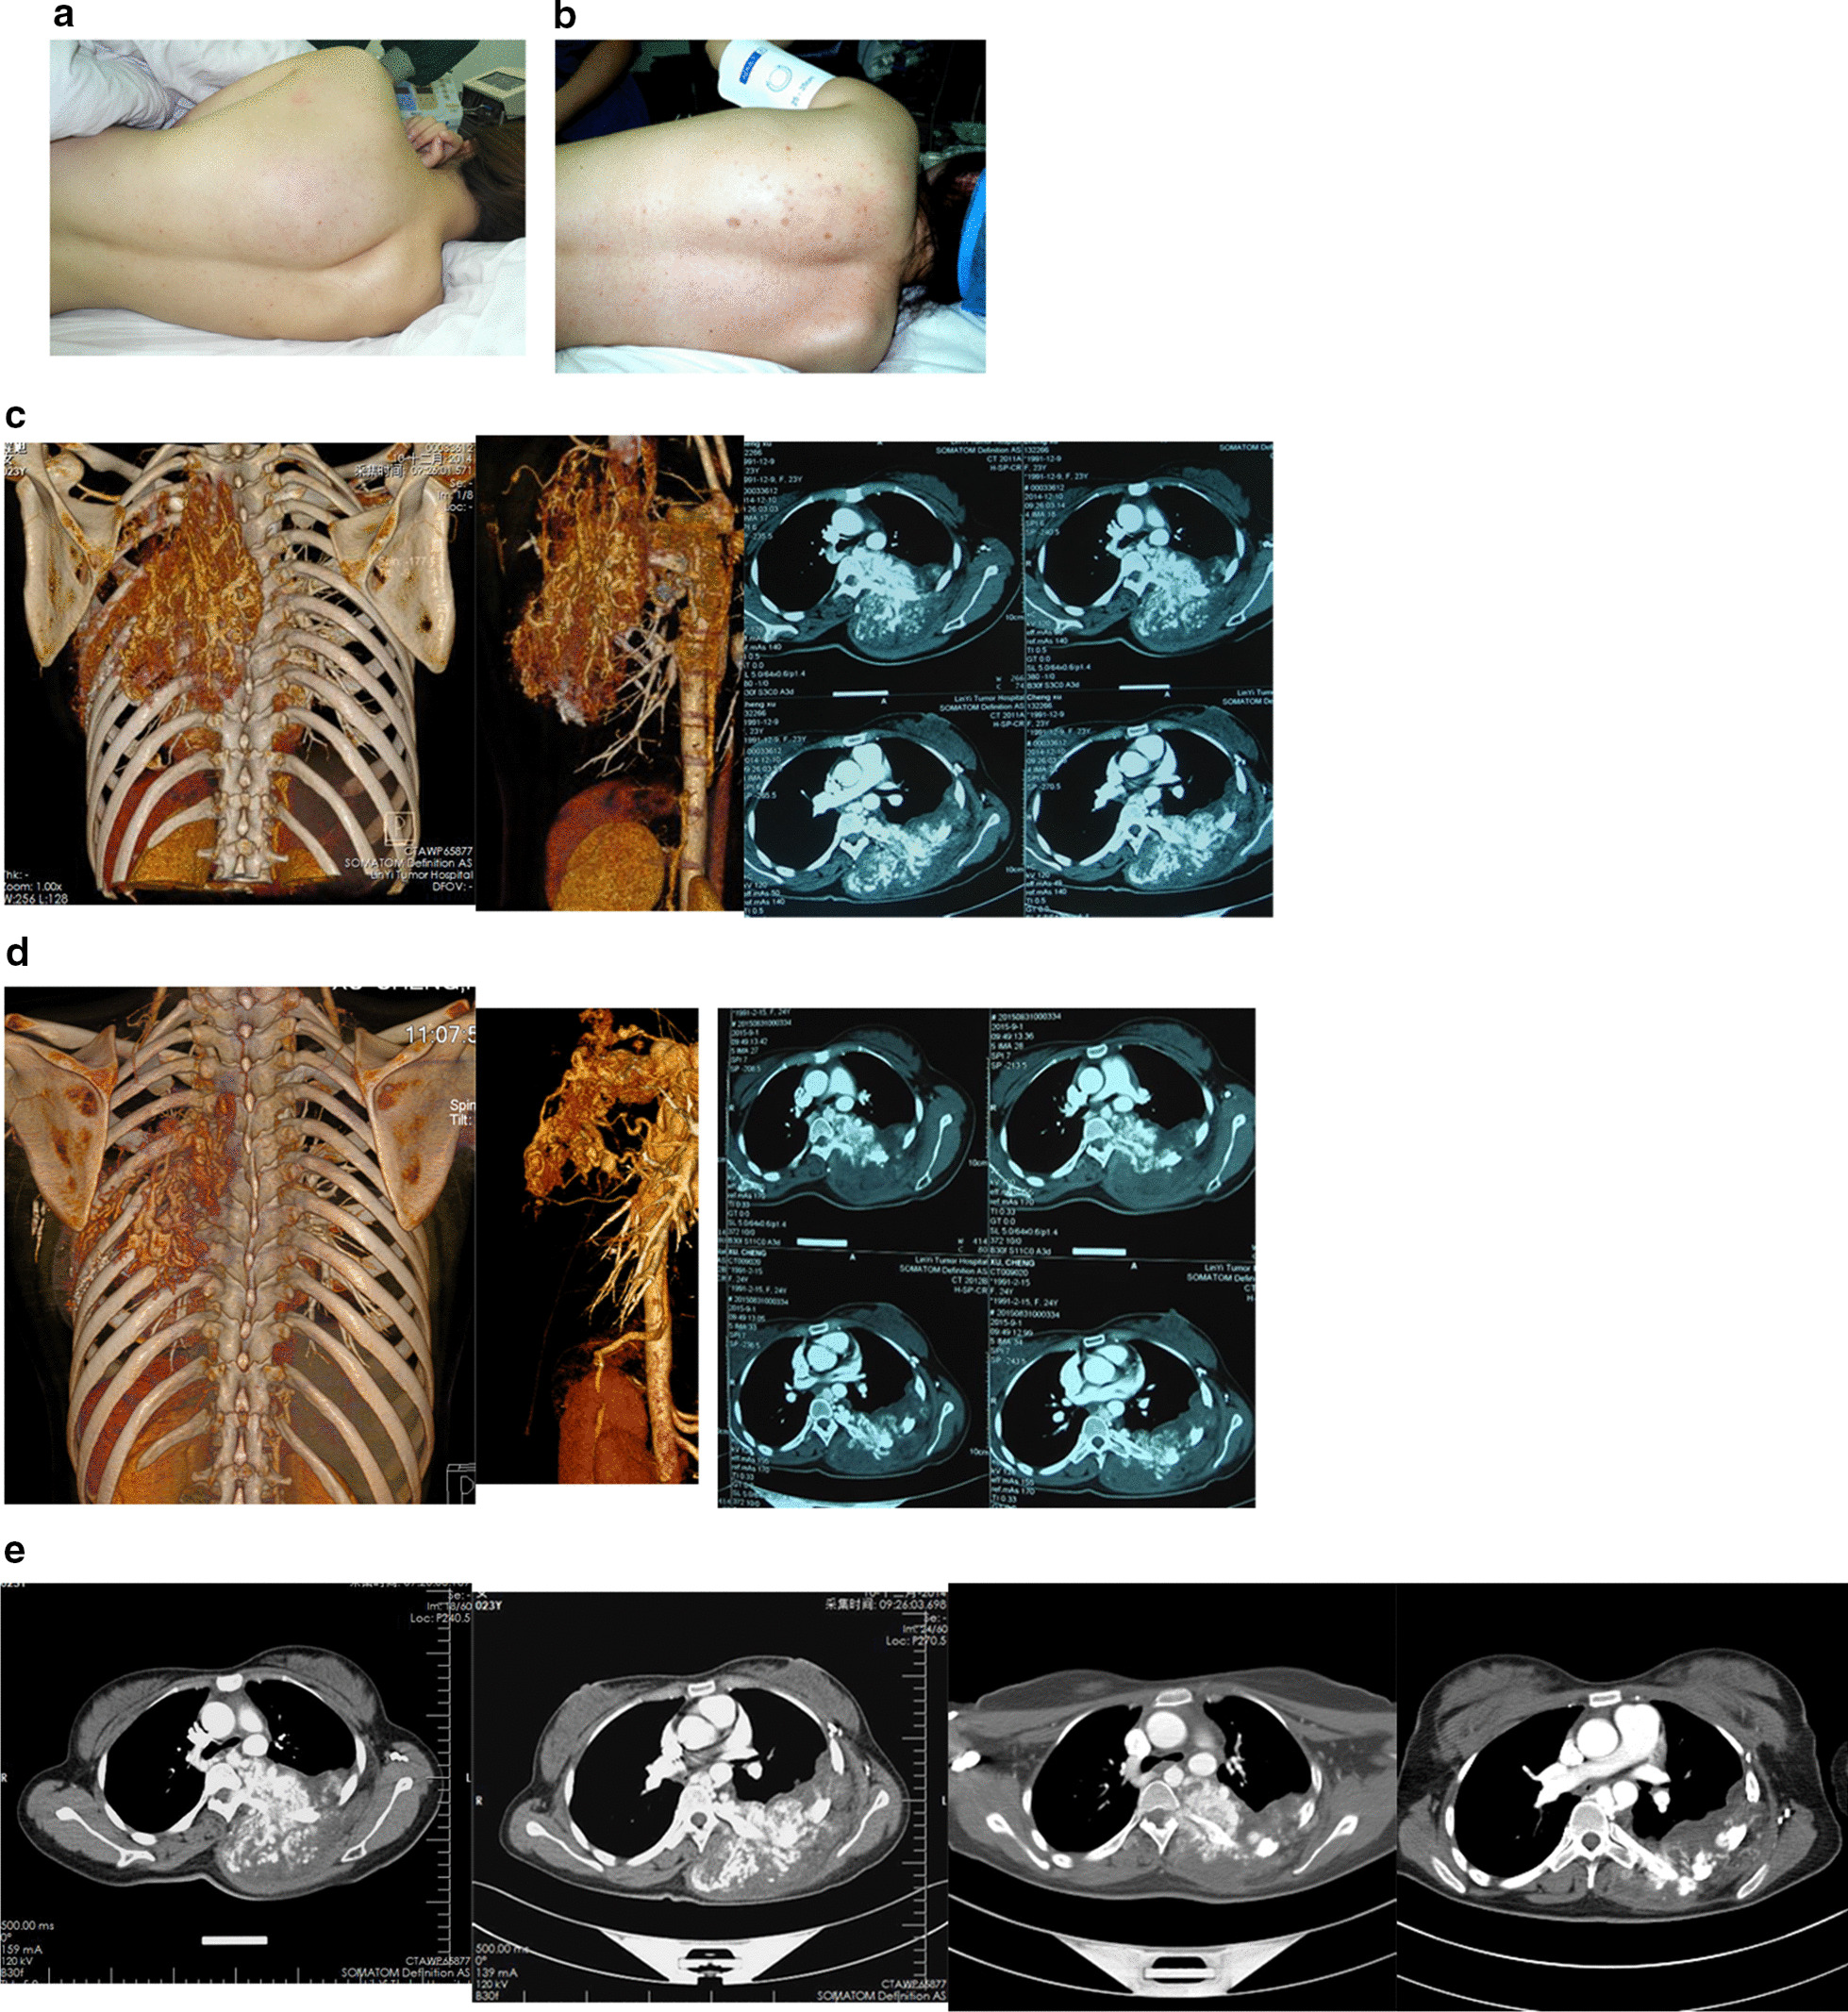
The comparison of diseased blood vessels and collateral vessels before and after treatment. a Before treatment, the lesion of the left chest and back was obviously raised and convex. b After treatment, the lesion of the left chest and back was obviously reduced, and multiple ablation needle marks were seen on the surface skin. c The pre-treatment CTA results showed that the lesion involved the left posterior chest wall, bone destruction, and multiple ribs and thoracic vertebrae. The lesion was obviously protruded into the chest, multiple blood supply arteries were enlarged, directly from the thoracic aorta, the collateral vessels were extremely abundant, and the lesions were obviously enhanced. d After treatment, the left erector spine lesions and intercostal muscles present in the CTA were significantly reduced and thinned. Most of the original enhanced lesions showed a low density, and the blood supply and collateral vessel number decreased significantly. e The CTA case comparison before and after treatment (3-year reexamination) showed that the left erector spine lesions and intercostal muscles had been further reduced and thinned. Most of the original enhanced lesions showed a low density, and the blood supply arteries and collateral vessels decreased significantly
